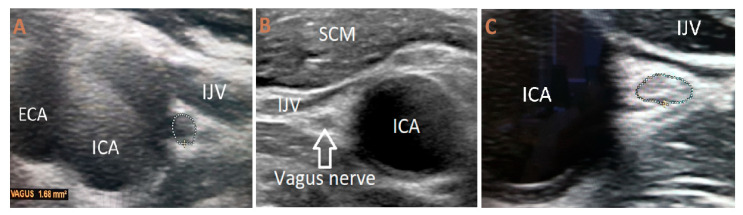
(A) Ultrasonography of the vagus nerve (USVN) showing a hypoechoic and homogeneous structure in a healthy volunteer. (B) USVN of a PD patient demonstrating an isoechoic and homogeneous appearance (C) USVN of a PD patient, demonstrating a hyperechoic and heterogenous appearance. The dotted circle indicates the cross-sectional area of the vagus nerve. SCM—sternocleidomastoid muscle; IJV—internal jugular vein; ICA—internal carotid artery; ECA—external carotid artery.
